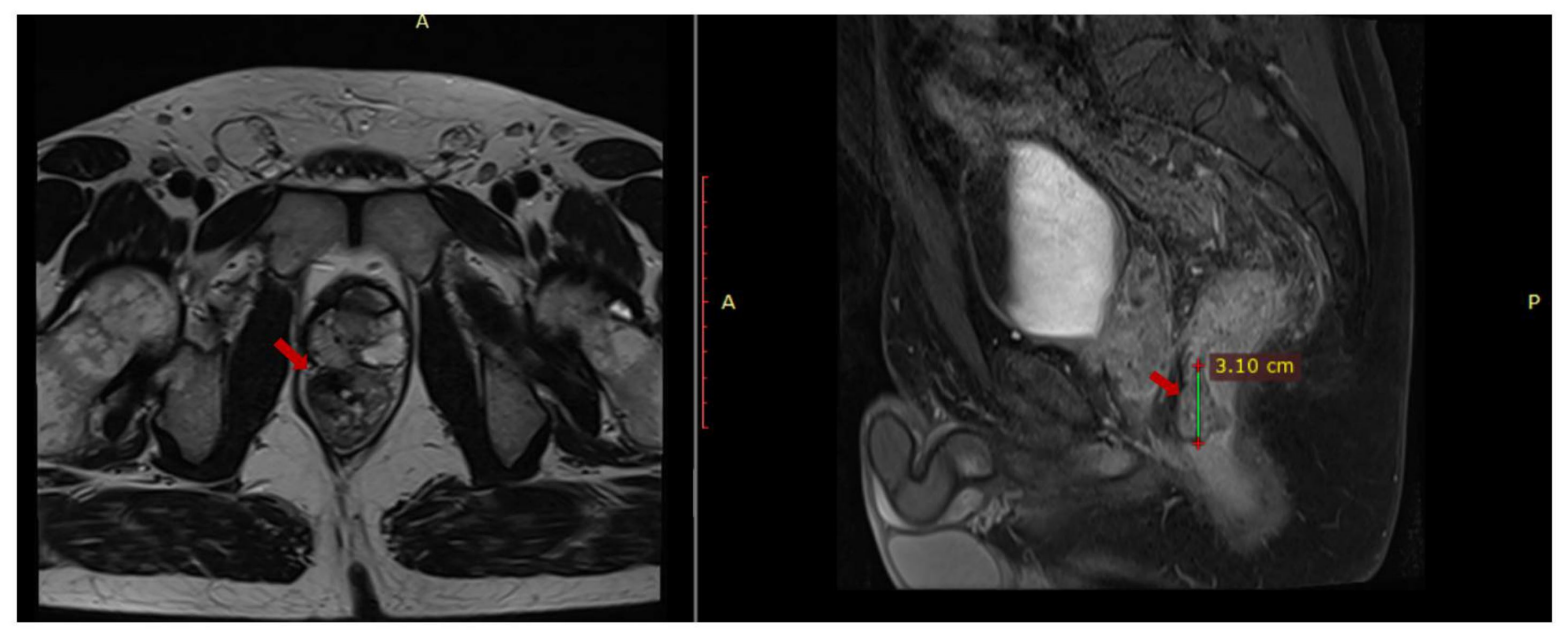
Pelvic MRI axial T1 and sagittal T2 sequences depict contrast-enhanced fibrous tissue in the rectum that adheres to the prostate.
